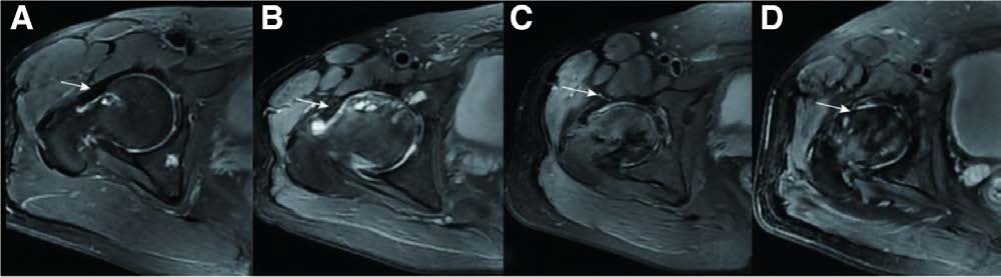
MRI classification of morphological alterations of the anterior capsule. (A) Grade 0, regular capsule without a hybrid signal in stage Ⅰ; (B) grade 1, local dotted high signal within the anterior capsule (edema) in stage Ⅳ; (C) grade 2, linear high signal within the anterior capsule but sustaining integrity (delamination) in stage Ⅱ; (D) grade 3, continuity of the anterior capsule disrupted and confused the boundary in stage Ⅲ. MRI = magnetic resonance imaging.
